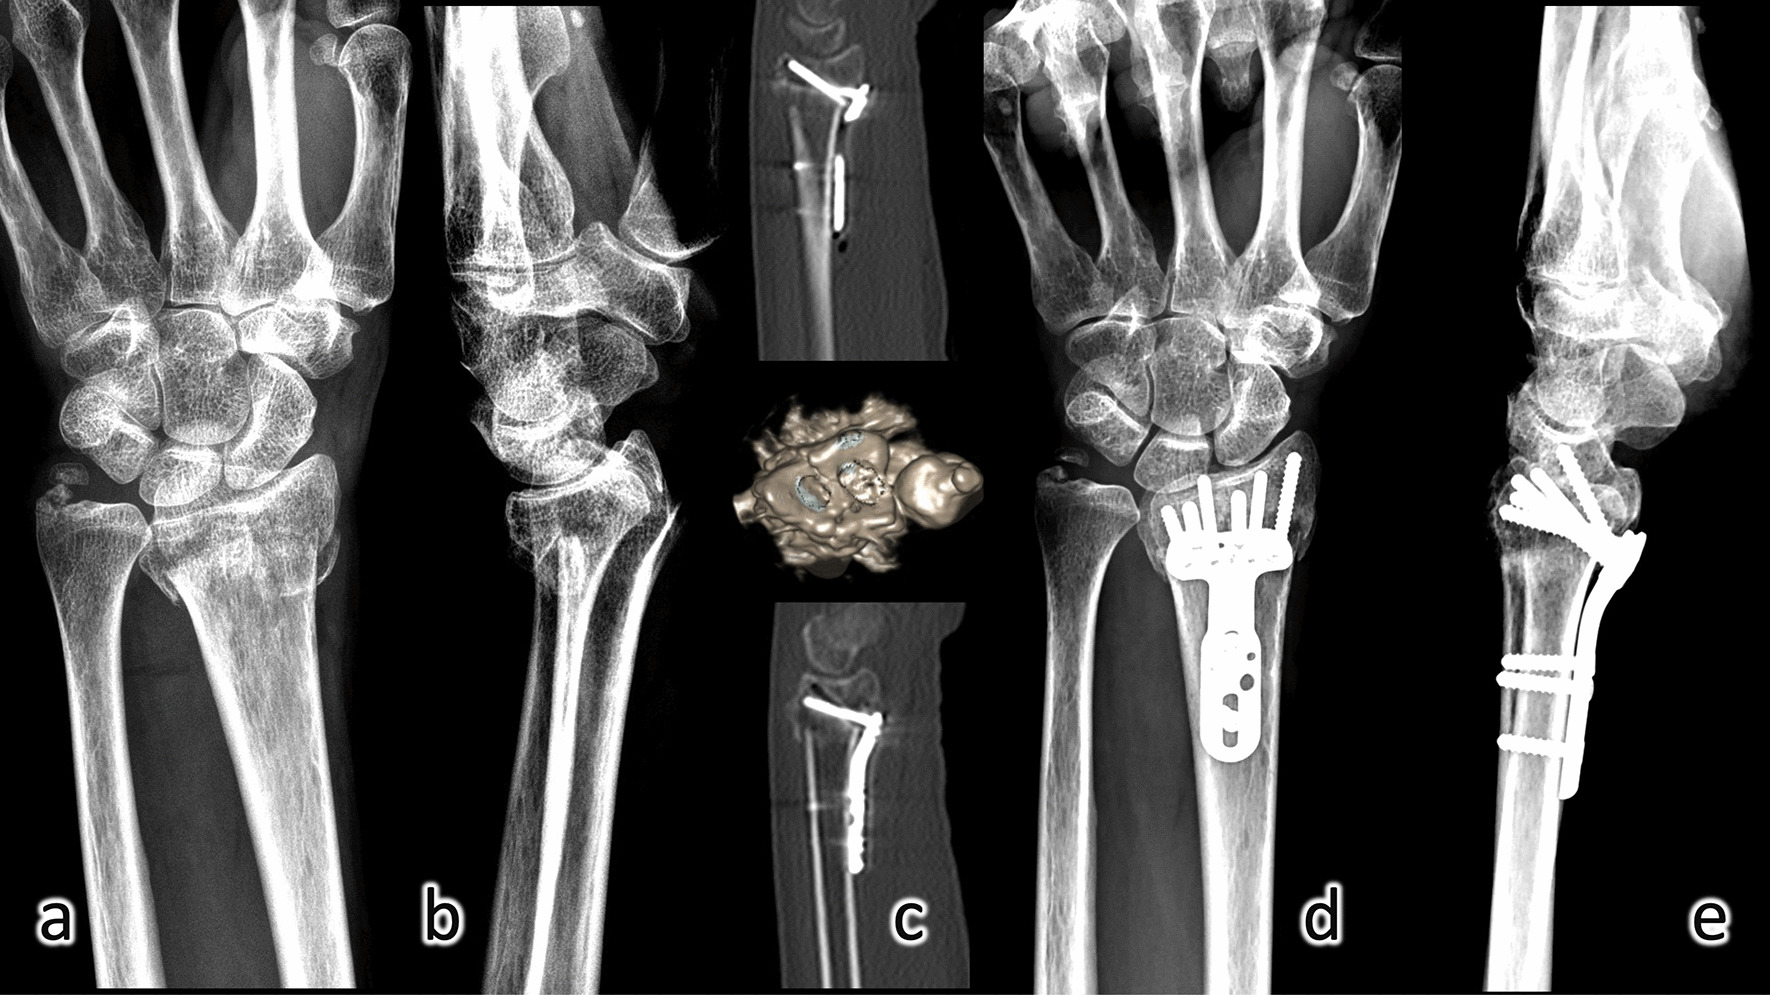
Medical images of a 61-year-old woman in radiograph of a posteroanterior view and b lateral view before operation; c postoperative computed tomography showed the screws captured comminuted dorsal ulnar corner fragment and anatomical reduction of the articular surface at both radiocarpal surface and sigmoid notch; radiograph of d posteroanterior view and e lateral view at 1-year follow-up postoperatively
